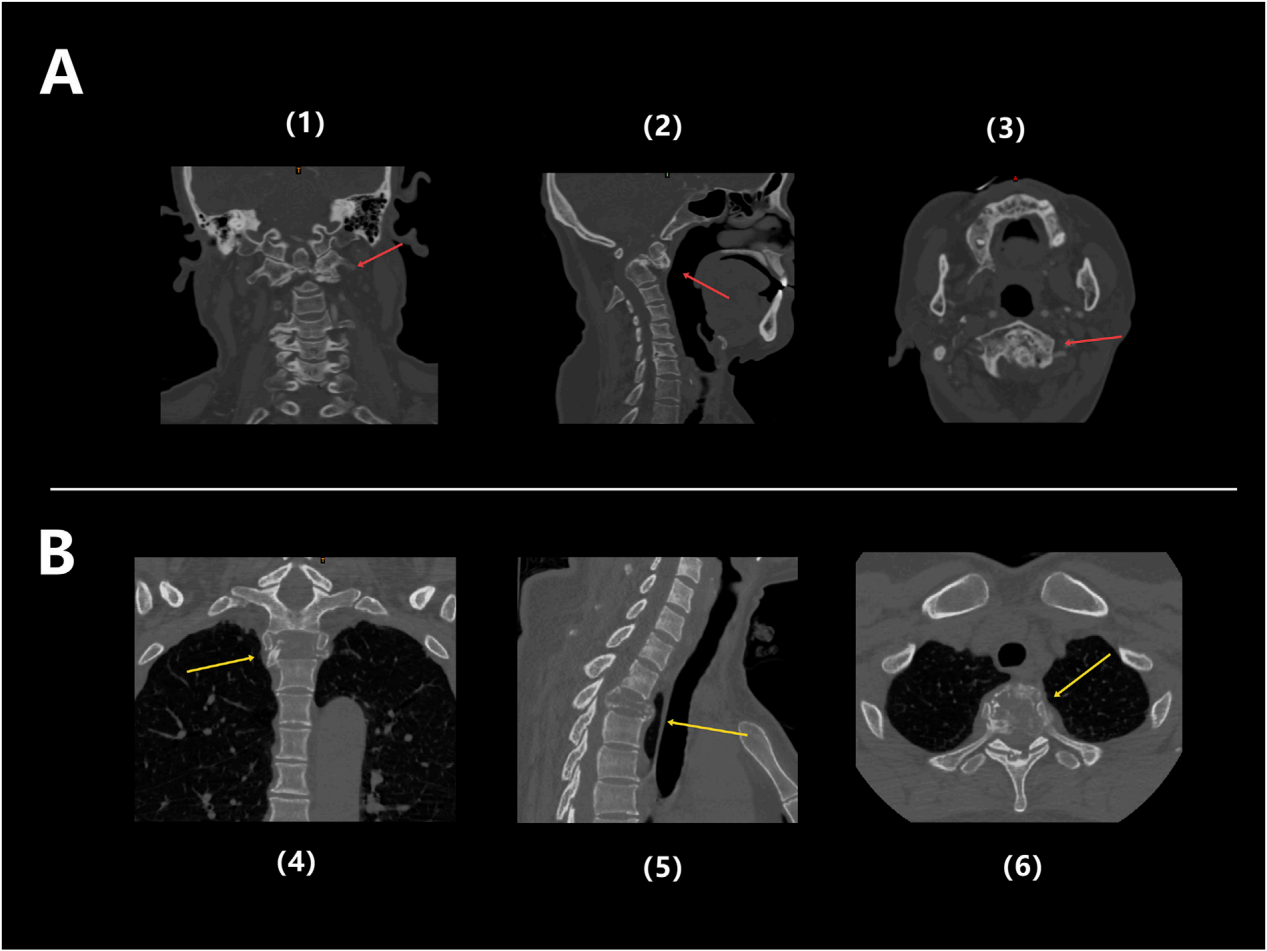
(A) Preoperative CT images of Patient 1: Coronal (1), Sagittal (2), and Axial (3), showing obvious structural abnormalities and irregular shapes in the atlantoaxial joint, with the backward tilted axis and significantly compressed and narrowed local spinal canal, and spinal cord compressed (red arrow); (B) Preoperative CT images of Patient 3: coronal (4), sagittal (5), and axial (6), showing significant bone destruction in the T2 thoracic vertebrae, accompanied by pathological fractures (yellow arrow).
